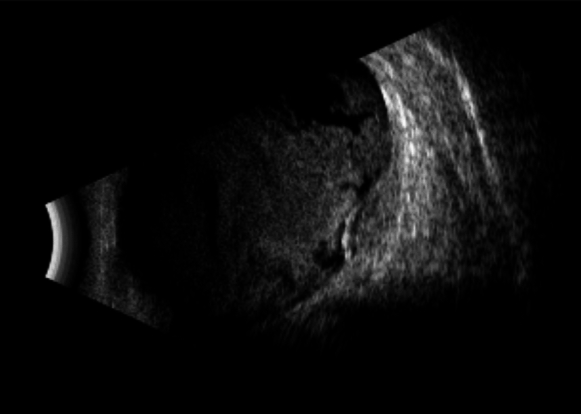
B-scan ultrasonography (US), showing a 2–3 mm thickened, heterogeneously layered submacular lesion with associated vitreous opacities, no signs of vitreoretinal traction. Findings suggest evolving age-related macular degeneration (AMD) and secondary vitreous opacities
